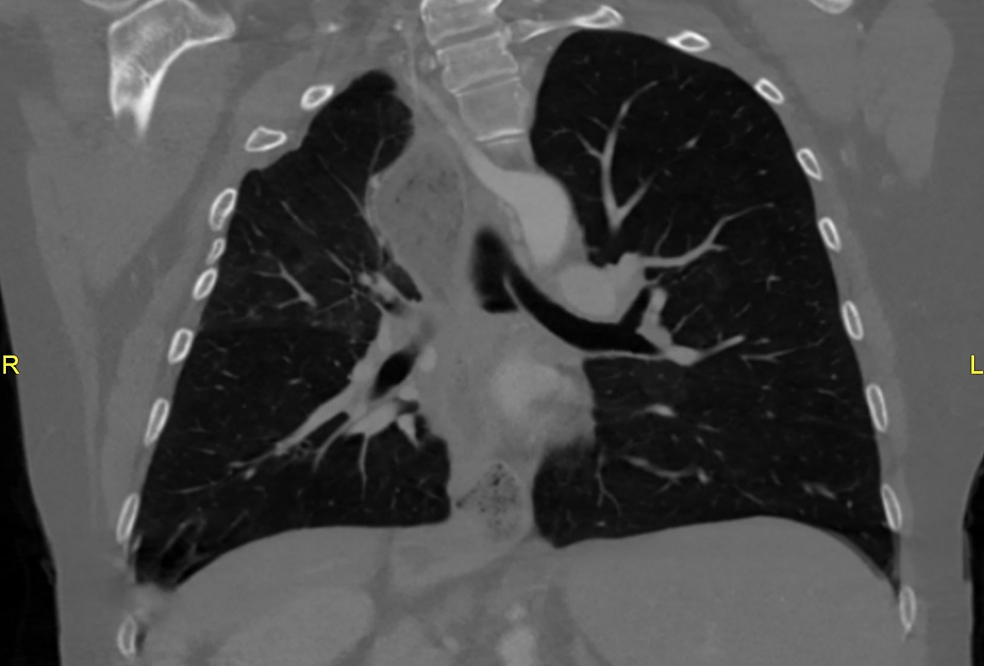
Coronal chest CT image demonstrating left vertical bronchus CT: computed tomography
